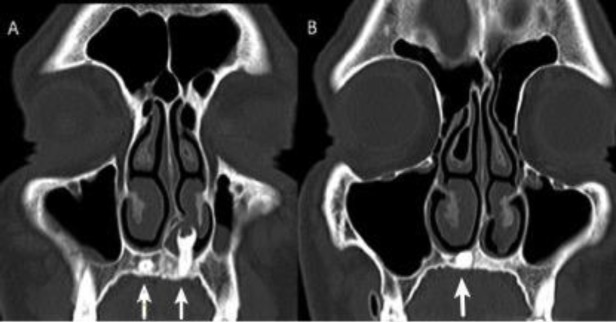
Coronal section of paranasal CT
